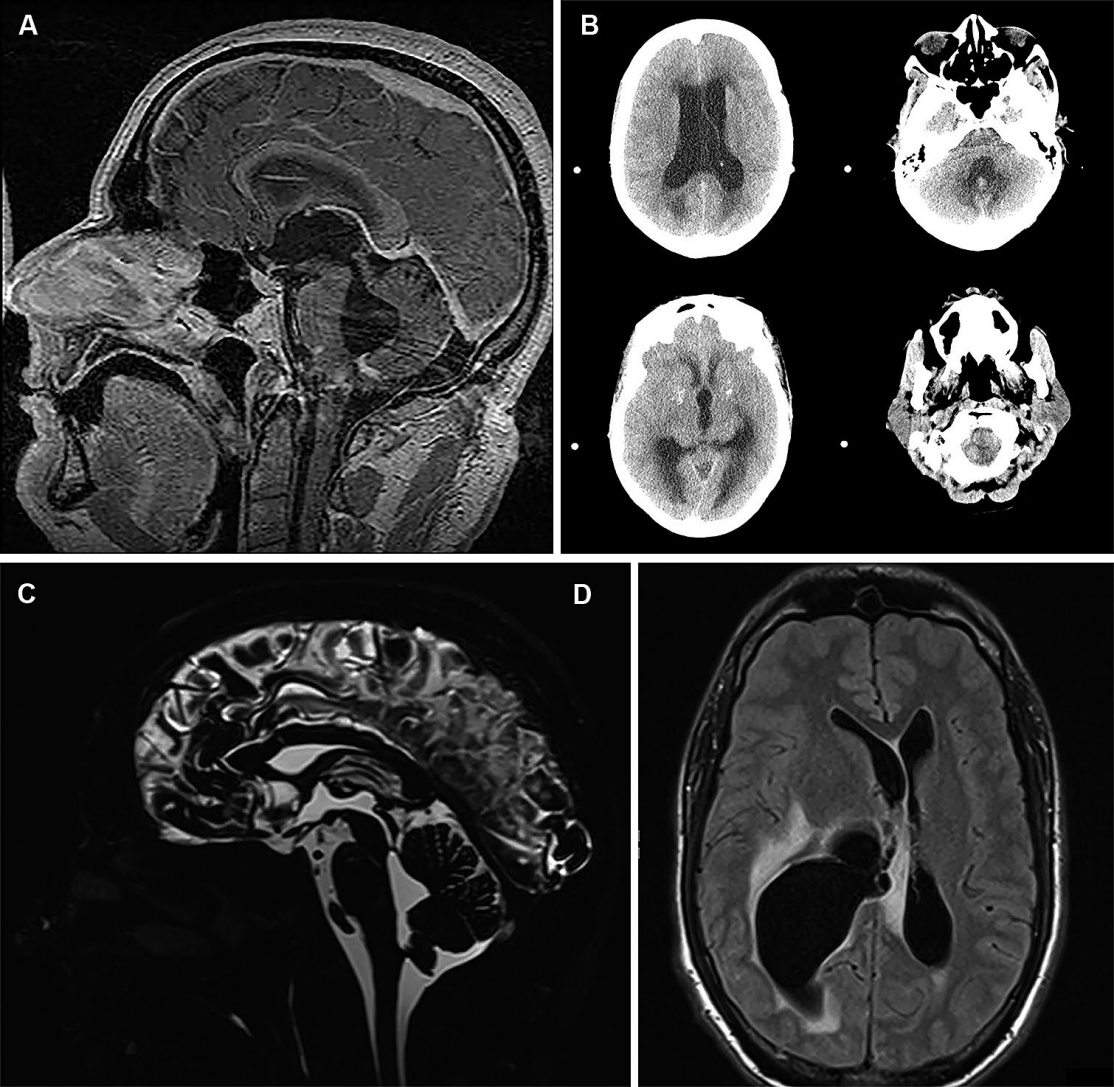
Obstructive hydrocephalus in neurosarcoidosis. Mid-sagittal contrast enhanced T1-weighted image (A) showing dilatation of the third and fourth ventricles and leptomeningeal enhancement of the bottom of the fourth ventricle. Axial CT scan (B) showing quadriventricular hydrocephalus and tonsillar herniation. Mid-sagittal T2-SPACE (C) showing a trapped fourth ventricle. Axial T2-FLAIR (D) showing dilatation of the right occipital horn due to a multicystic process located near the foramen of Monro
